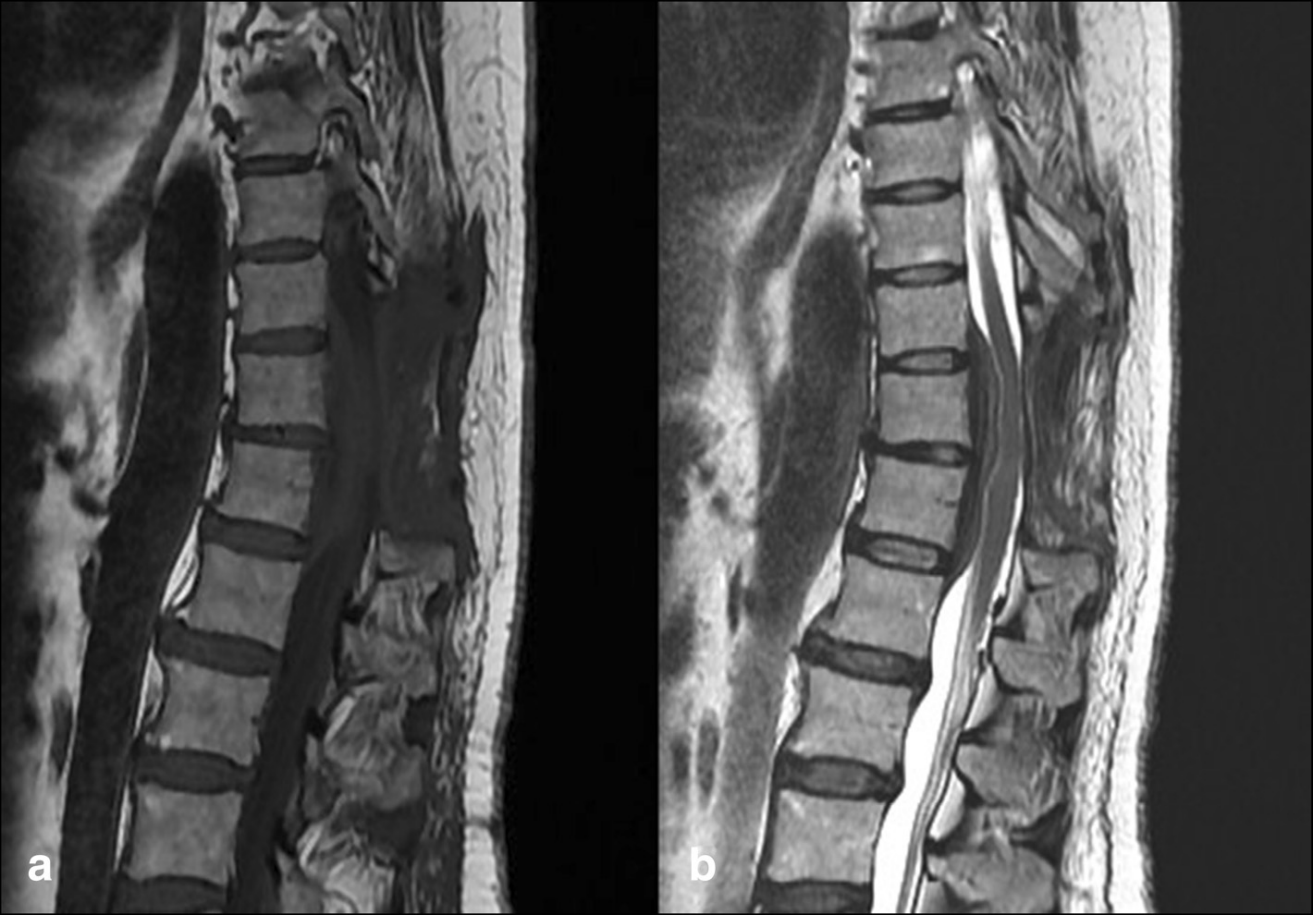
Gadolinium-enhanced magnetic resonance images 3 weeks after the first surgery showing regrowth of the epidural mass in the same localization. a T1 sagittal view. b T2 sagittal view
